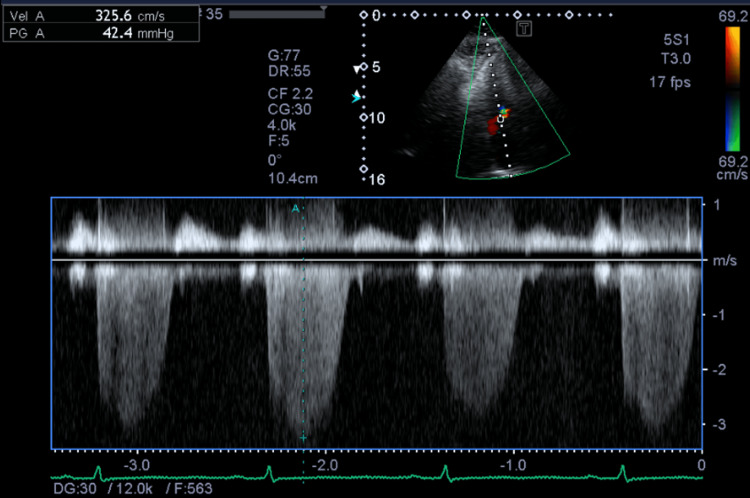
Echocardiographic Assessment of Pulmonary Hypertension The spectral Doppler trace demonstrates a peak velocity of 3.26 m/s, corresponding to a tricuspid regurgitation pressure gradient (TRPG) of 42.4 mmHg, indicating mild pulmonary hypertension.
